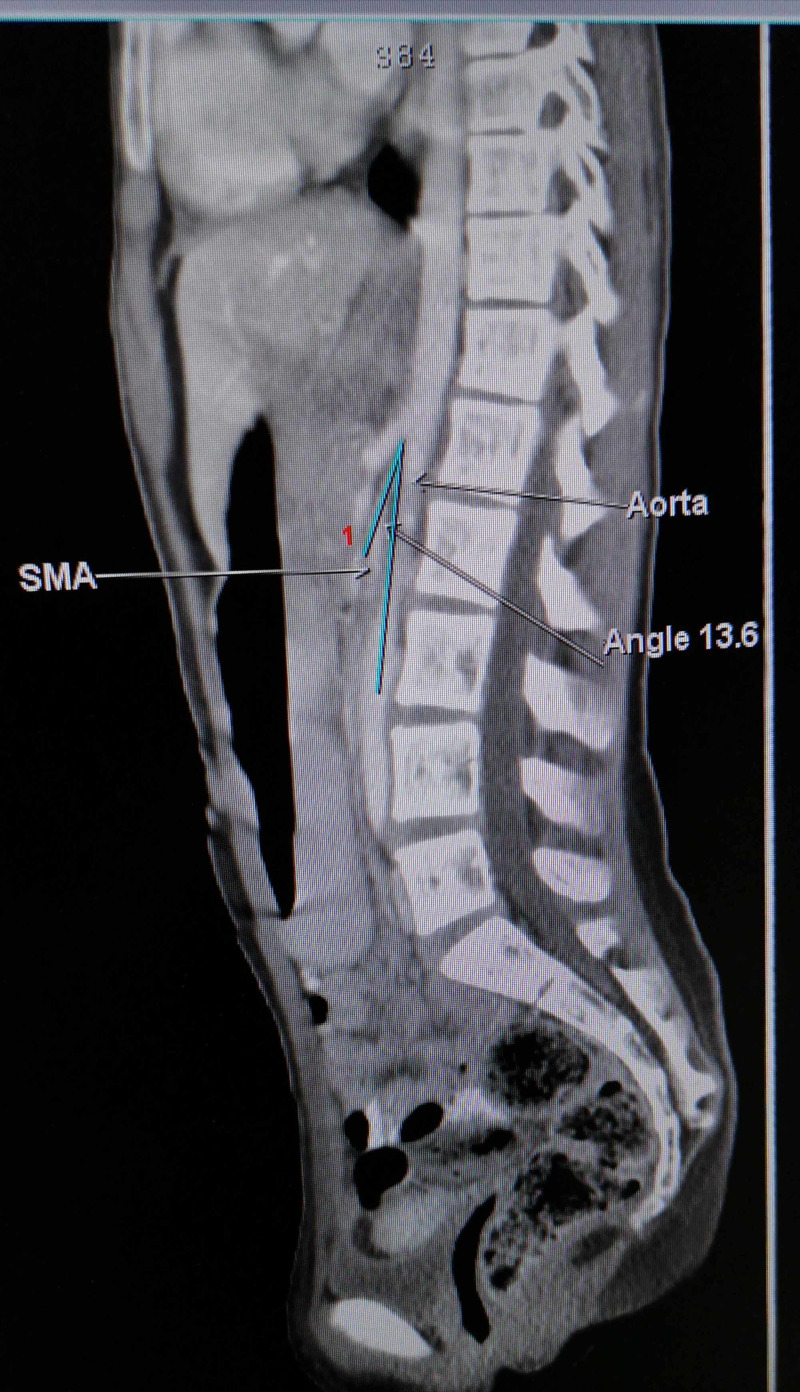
CT scan showing a reduced aortomesenteric angle, measuring 13.6° (normal 25°-60°), reaffirming the diagnosis of Wilkie’s syndrome. SMA: superior mesenteric artery
